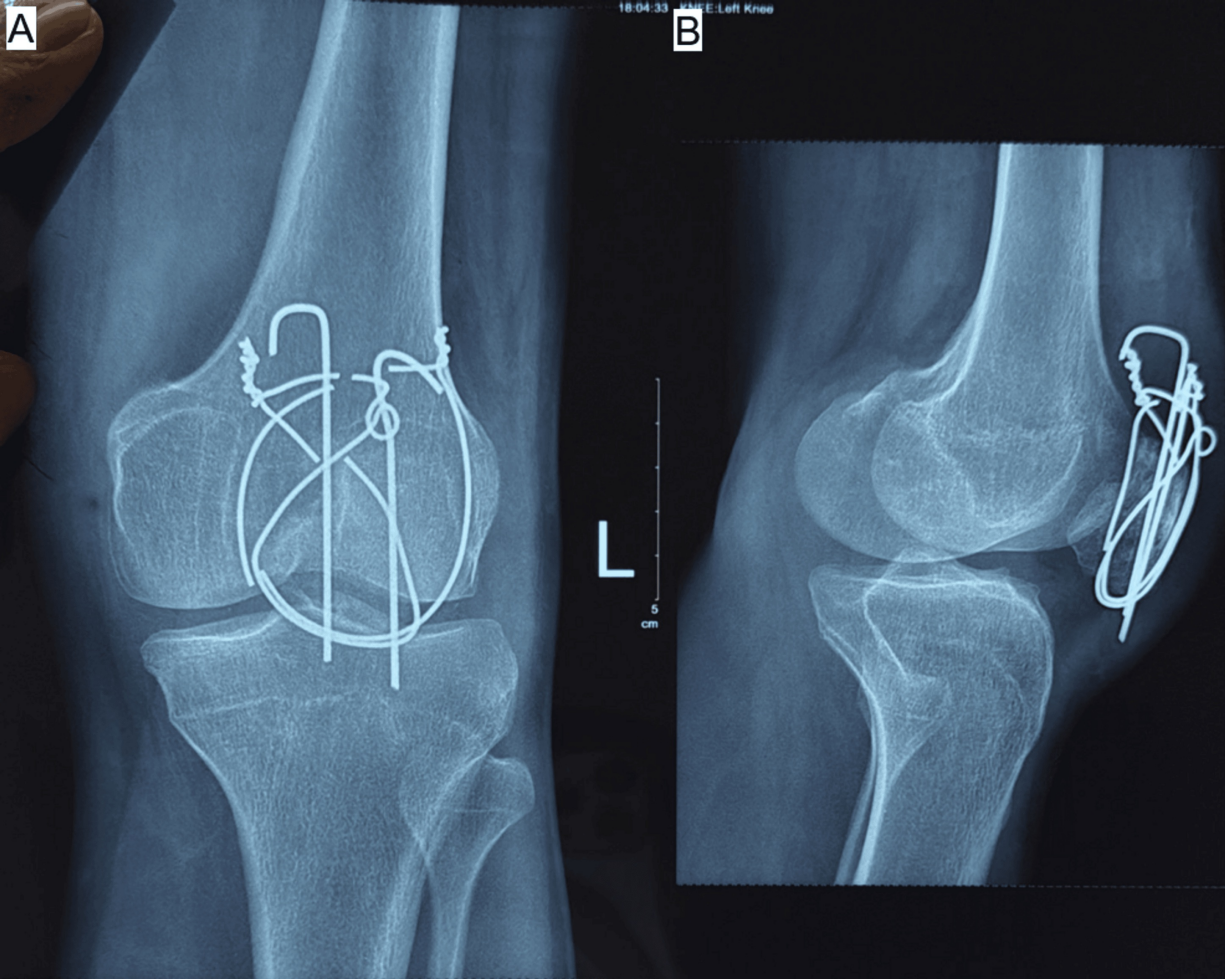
(A, B) Postoperative radiograph of TBW patella in transverse patella fracture at 6 months. Source: Images courtesy of the authors. TBW: tension band wiring
